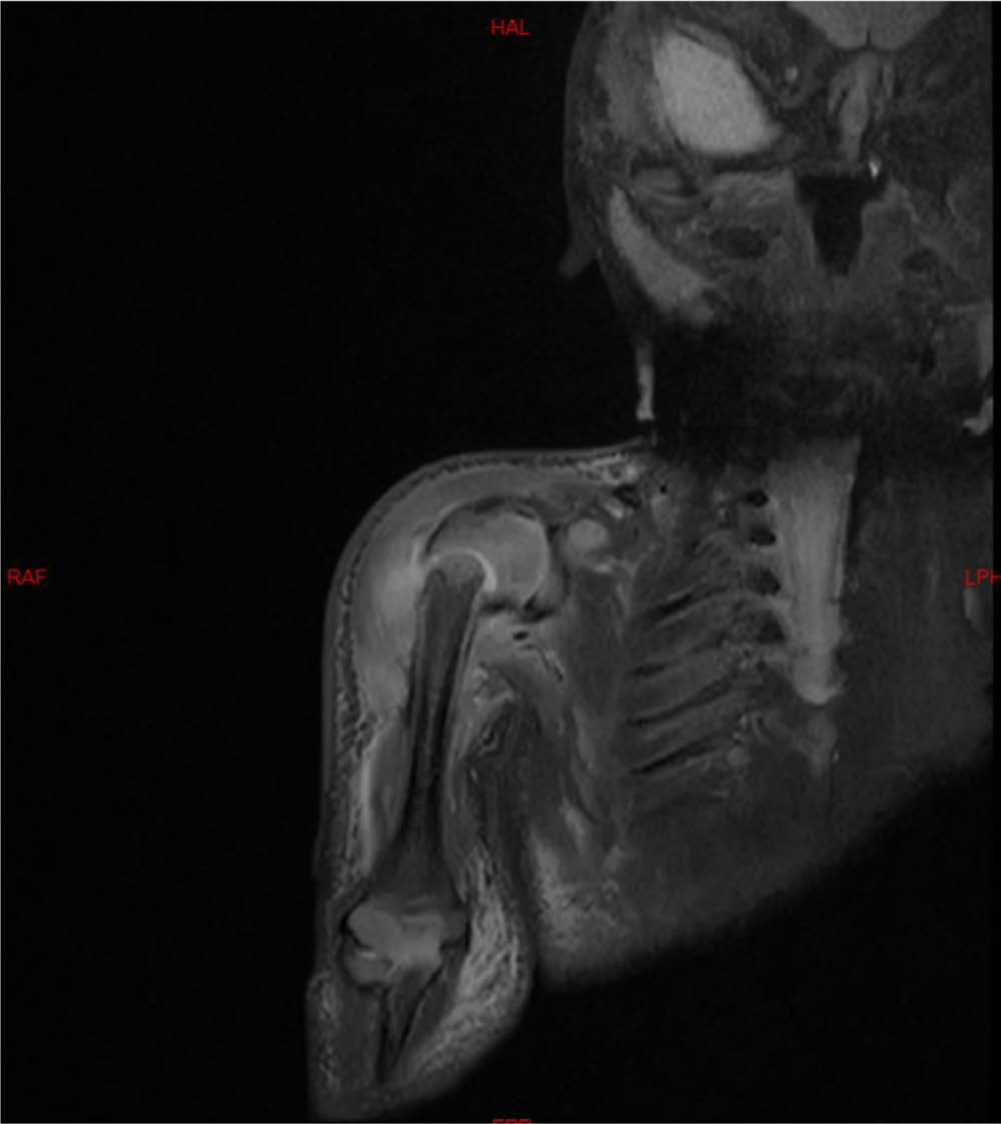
MRI of the right shoulder in a 12-day-old neonate (Case 2). Fracture of the right humeral head epiphysis with associated periarticular soft tissue injury and right shoulder joint effusion. MRI = magnetic resonance imaging.
